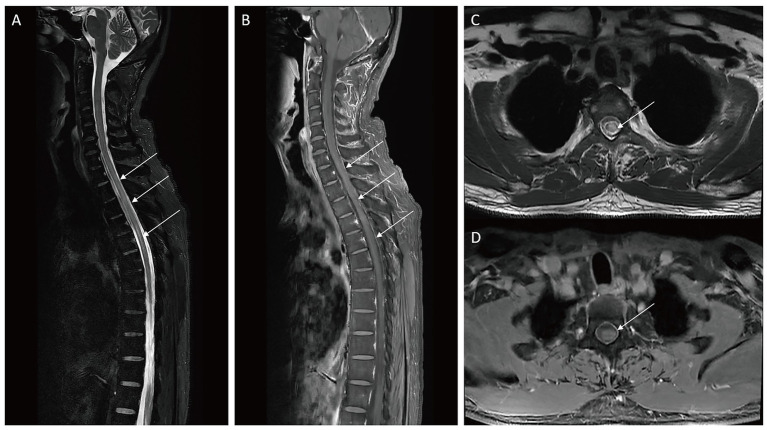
Spinal cord magnetic resonance imaging (MRI). (A) Sagittal T2 showing hyperintensities at T1–T6 levels of the spinal cord (arrows). (B) Sagittal T1 gadolinium enhancement showing mild enhanced signals at the level of T1–T6 (arrows). (C) Axial T2 sequence showing hyperintense lesion in the central part of the spinal cord (arrow). (D) Axial T1 gadolinium enhancement showing mild enhanced signal in the central part of the spinal cord (arrow).
